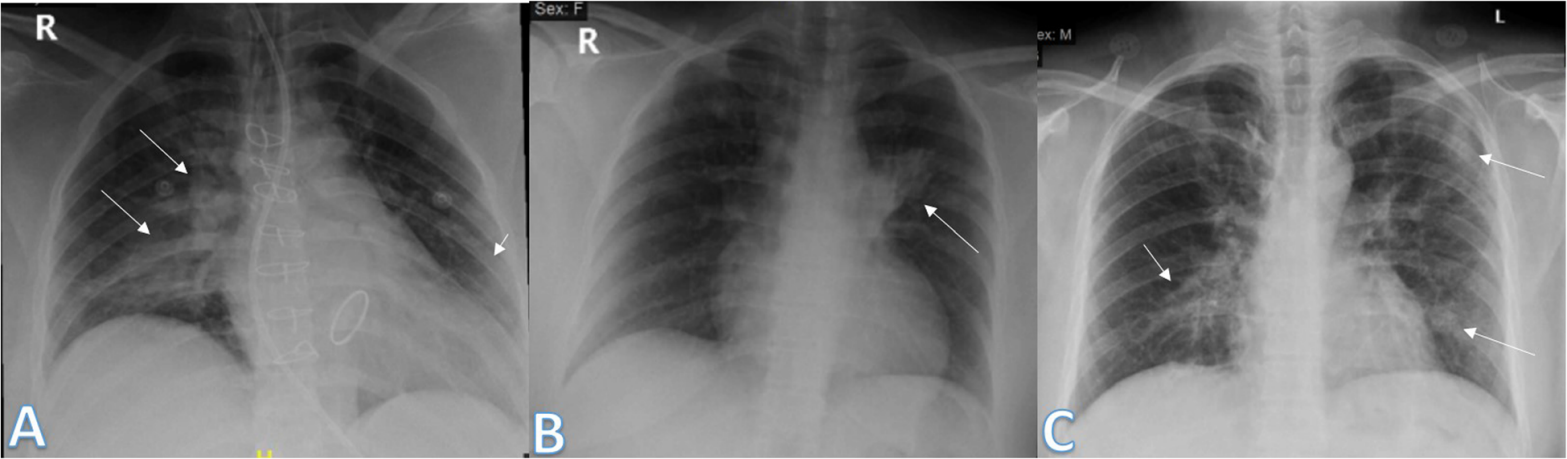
Three different patients with positive COVID-19. a 39-year female was complaining of SOB with tachypnea, fever, and cough. She had previous open cardiac surgery with valve replacement. She was intubated due to hypoxemia. Chest X-ray showed right perihilar air space consolidation opacity extending to the right paracardiac region (long arrows). Another left lower zonal air space consolidation opacity was seen (short arrow); the severity score was 2 for the right lung and 1 for the left lung, so TSS was 3. b 44-year female was complaining of fever. Initial chest X-ray showed left perihilar air space consolidation opacity (arrow). The total severity score was 1. c 35-year male was complaining of cough and fever. Initial chest X-ray showed right perihilar air space consolidation opacity (short arrow). Other left lower zonal air space consolidation opacities are seen (long arrows) with reticular thickening; the severity score was 1 for each lung, so TSS was 2
